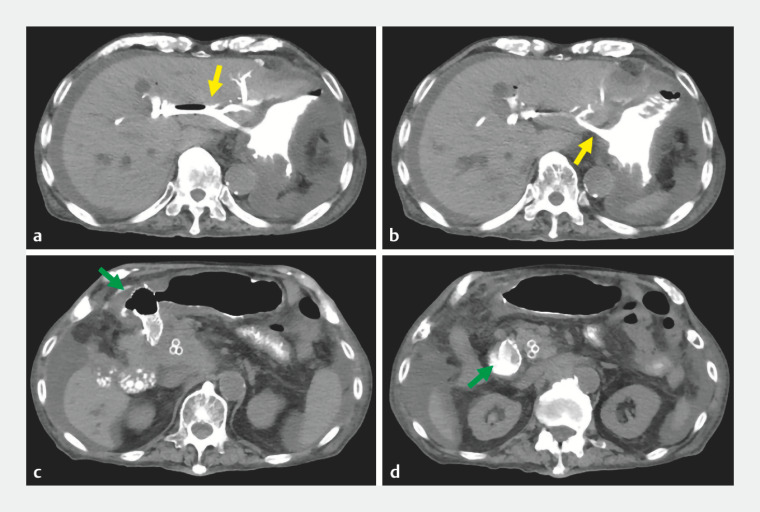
Axial CT immediately after EUS-HGS and enteral stenting:
